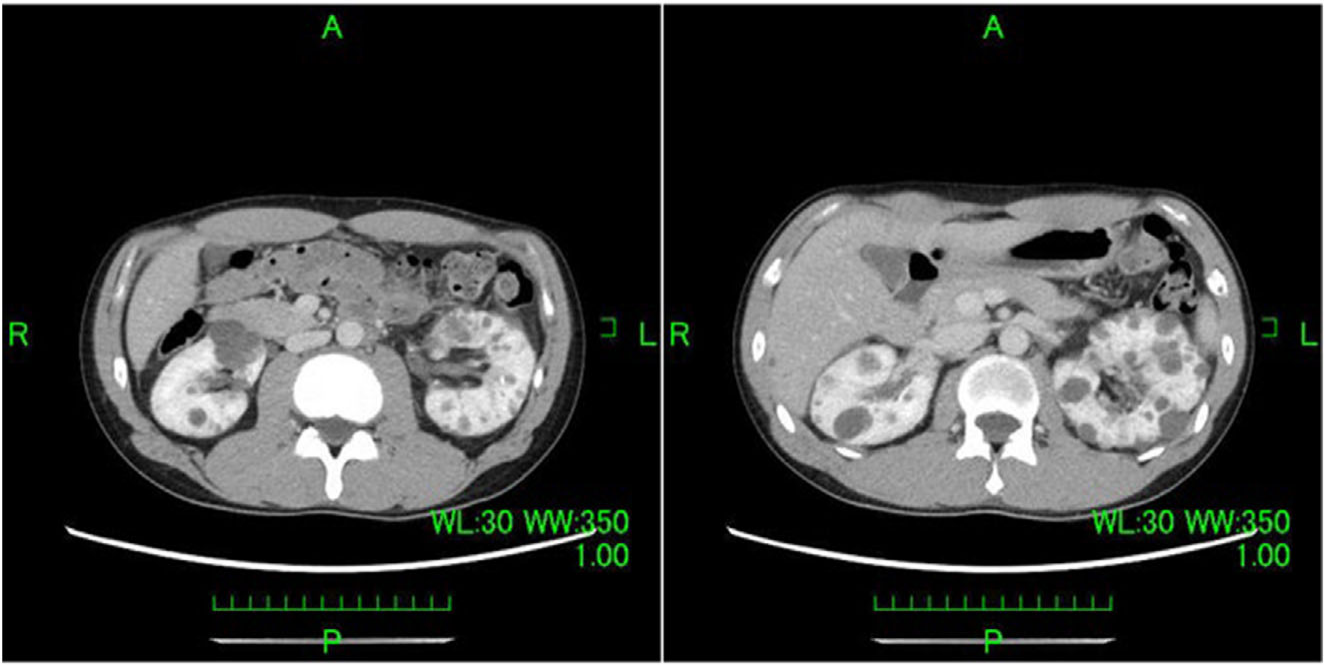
Six months after treatment initiation, a CT evaluation revealed that the mass lesion in the left renal parenchyma that extended to the renal pelvis and para‐aortic lymph nodes was smaller.
